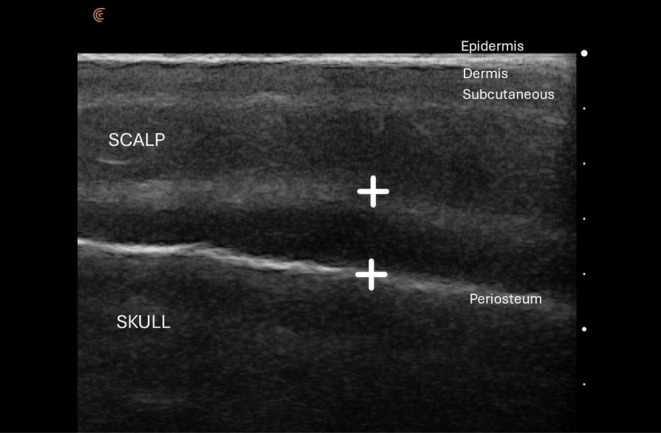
Six‐month later ultrasound picture showing layer where Lanluma V was injected over the galea.
